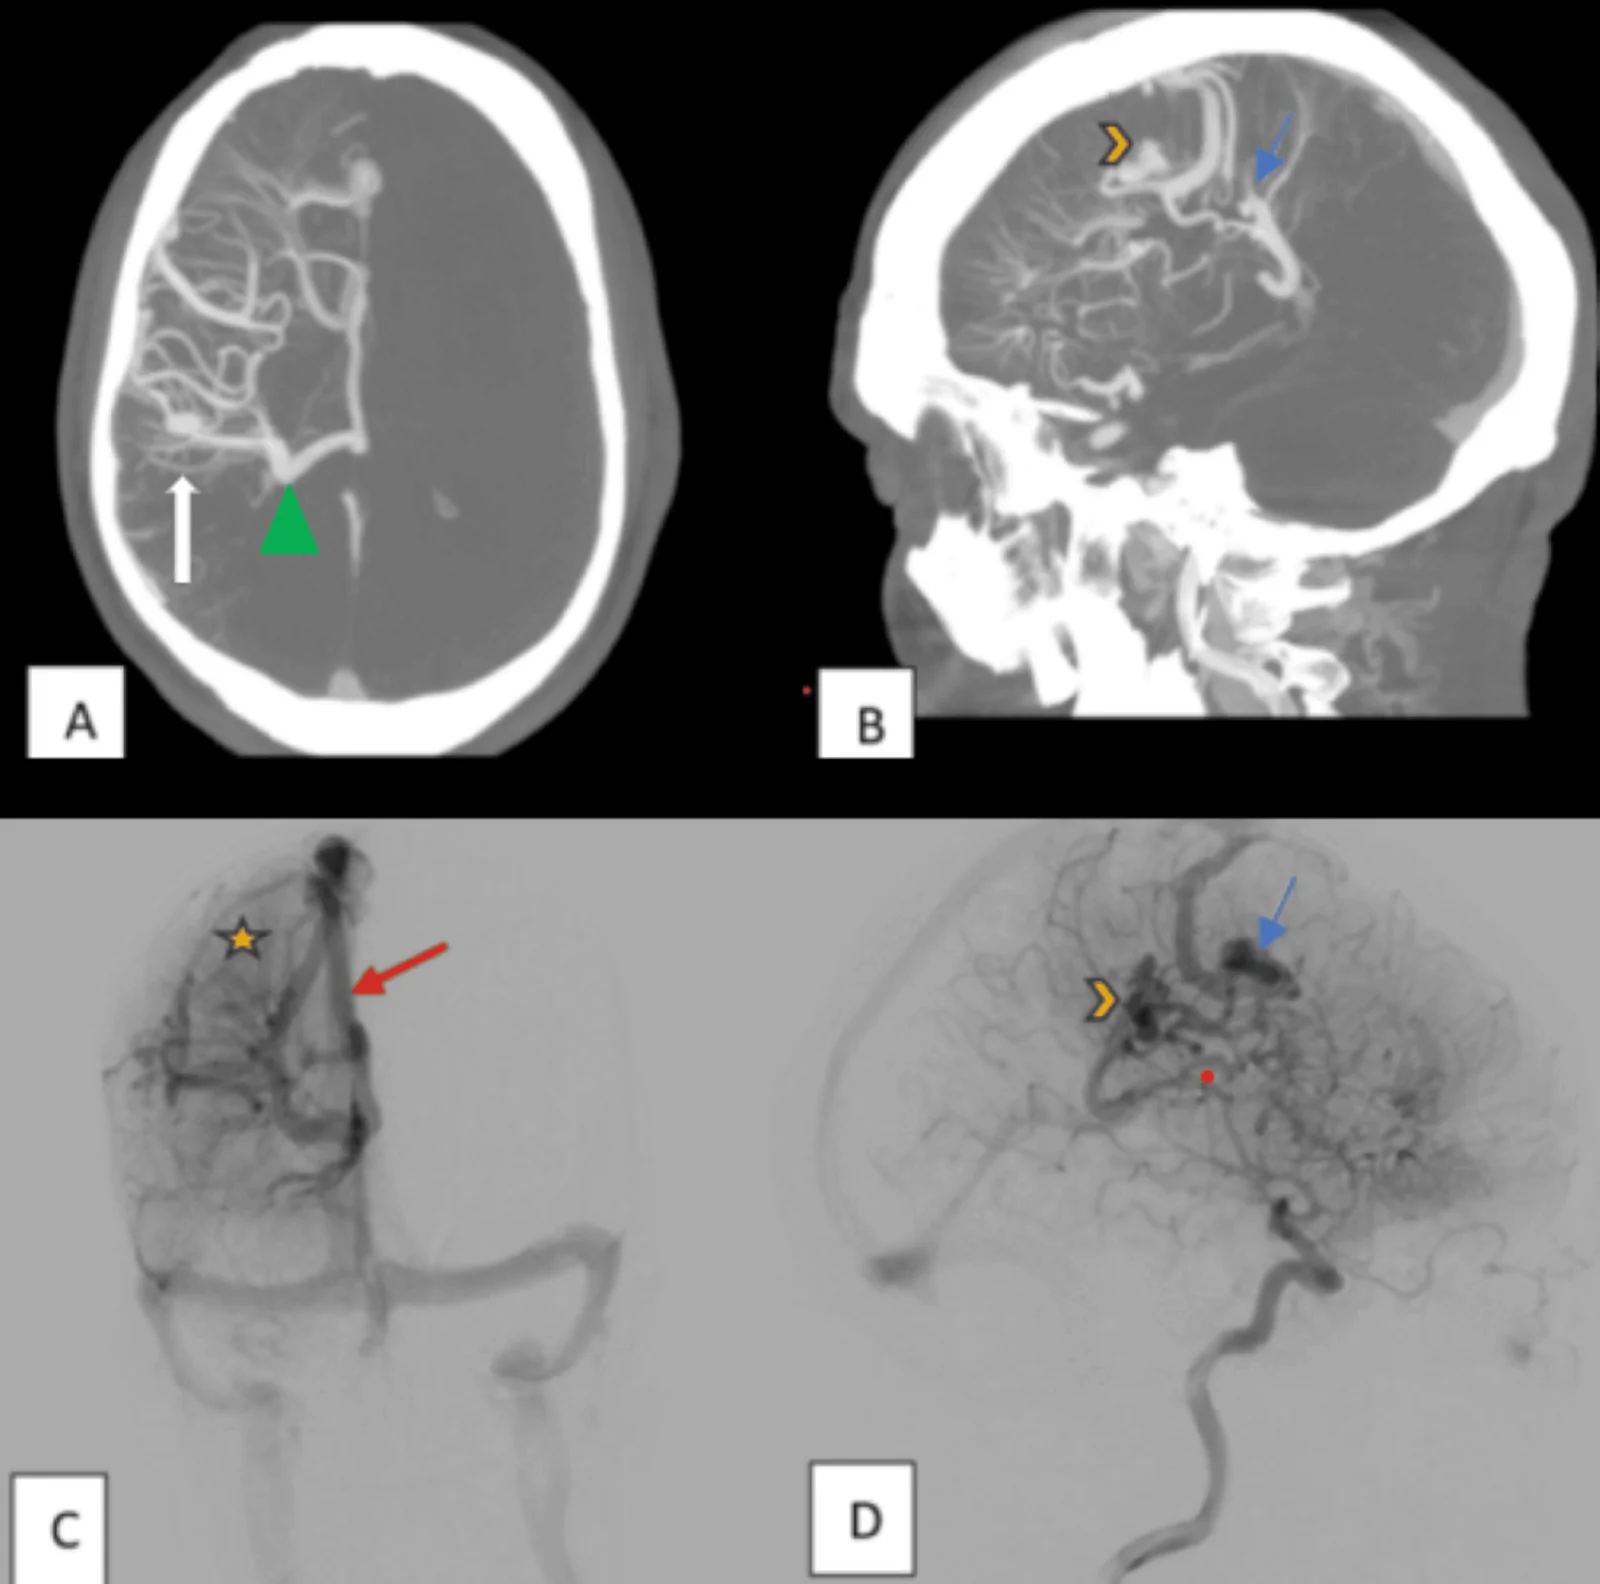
3D reconstructed CT angiograms (A, B) demonstrating two right-sided AVMs located in the frontal (head arrow) and paracentral (blue arrow) regions In image A, the afferent arteries (white arrow) and the draining vein (green arrowhead) of the paracentral AVM are depicted. Cerebral angiography in anteroposterior (C) and oblique (D) projections shows multiple hemispheric radial veins converging into a dilated cortical collector vein and draining into the superior sagittal sinus (red arrow), creating the characteristic 'caput medusae' appearance (star) of a DVA. AVM: arteriovenous malformation; DVA: developmental venous anomaly
